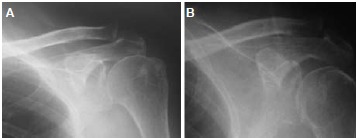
Fig. 2: T2-weighted MRI left shoulder (coronal view) showing edema pattern beneath the left acromioclavicular joint (arrow) with arthritic changes. F2:
